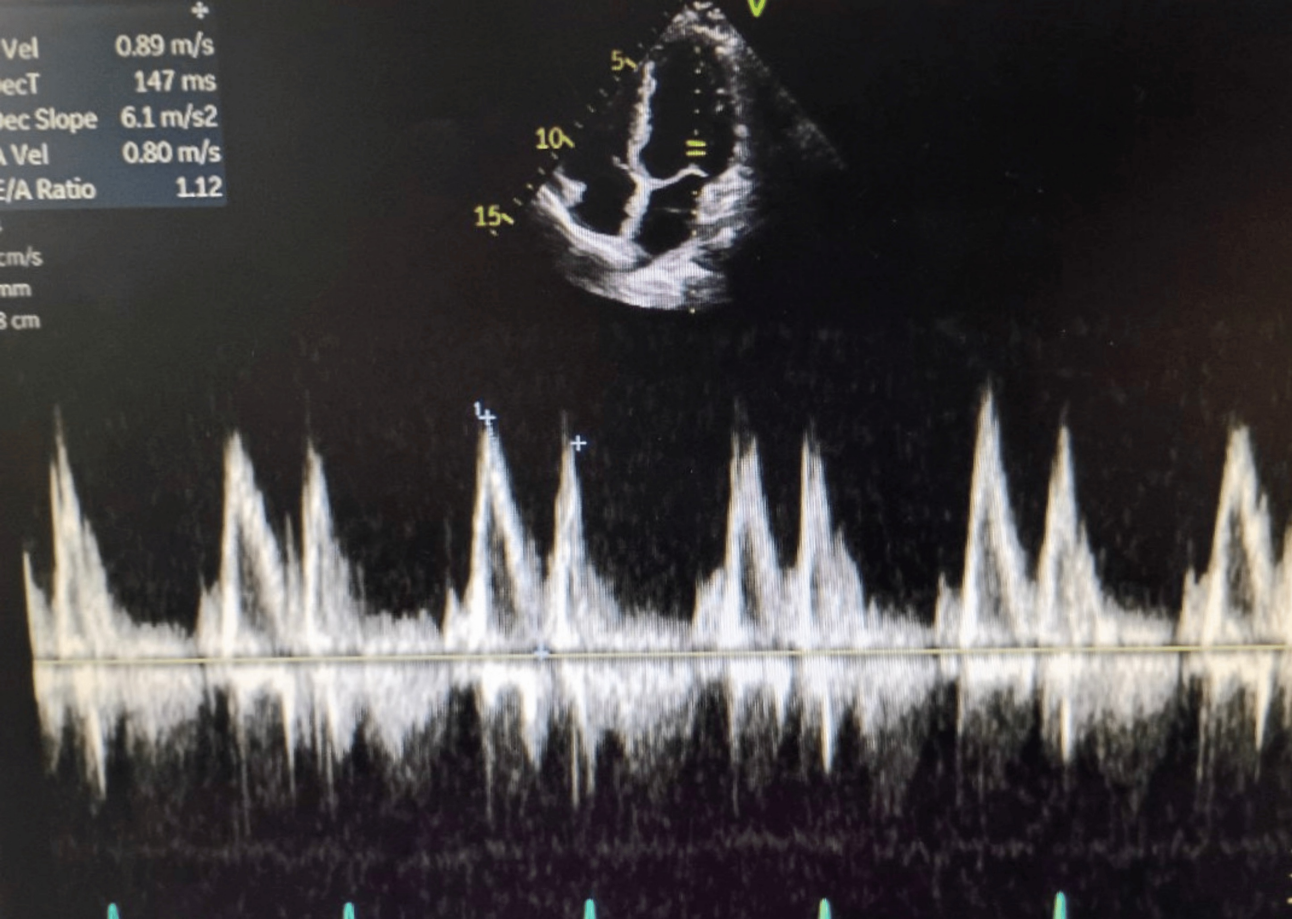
Echocardiogram showing a normal left ventricular (LV) diastolic function.
